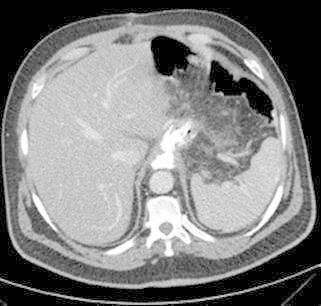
Abdominal CT-Scan with contrast leak
